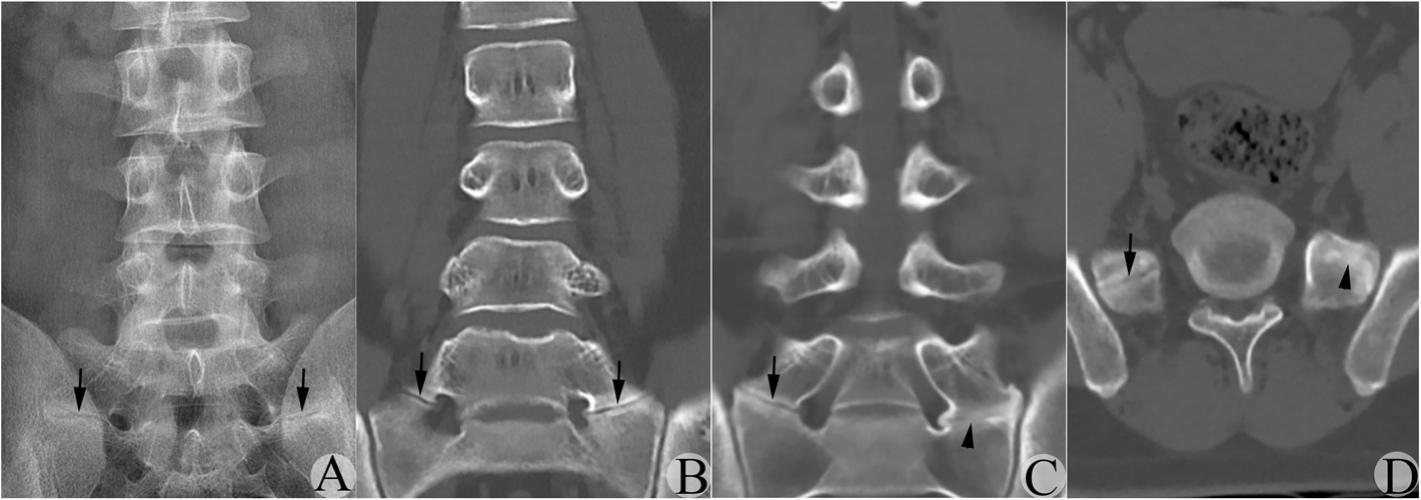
A suspected type IIb MA-LSTV judged by AP-LPR was verified to be type IV by CT-CRI. a AP-LPR image showing the suspected JLS between the TP of the MA-LSTV and sacrum bilaterally. Arrow indicates the suspected JLS. b CT-CRI showing the JLS was found in most CT-CRIs bilaterally. Arrow indicates the JLS. c CT-CRI showing the detected BUS on left side in only a few CT-CRIs through the posterior vertebral body. Arrow indicates the JLS; arrowhead indicates the BUS. d Transverse CT image verified the JLS on the right side, while the BUS was on the left side. Arrow indicates the JLS; arrowhead indicates the BUS
